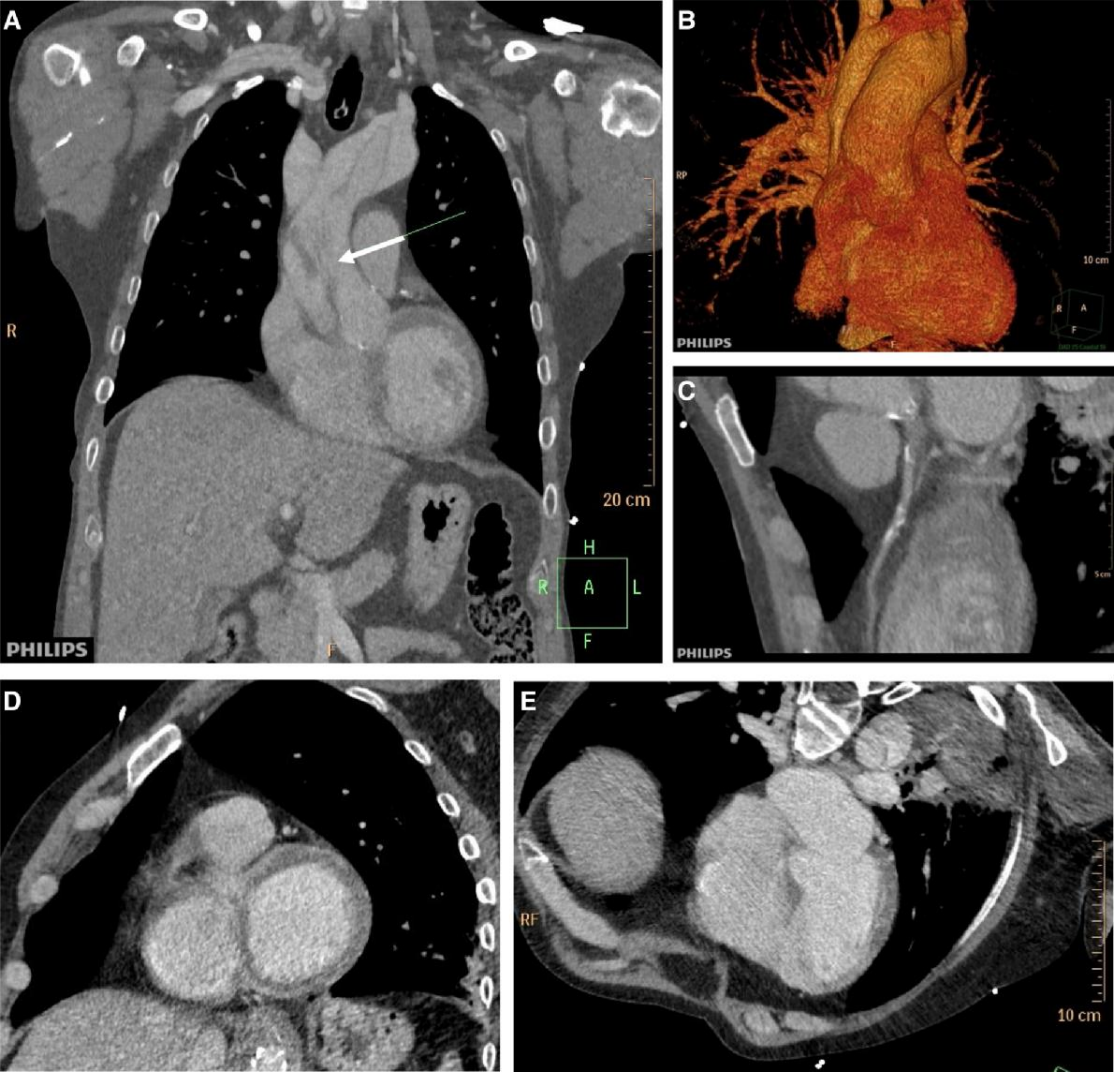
Chest computed tomography angiography showing acute Stanford Type A aortic dissection. (*A*) Coronal projection shows the entry tear in the ascending aorta (white arrow). (*B*) Volume rendering and (*C*) left coronary artery with calcified plaques. (*D* and *E*) Oblique sagittal and axial projections showing myocardial perfusion defects. Because of the poor quality of the study, the mechanism by which the left-main coronary ostium was involved in this case could not be described.
